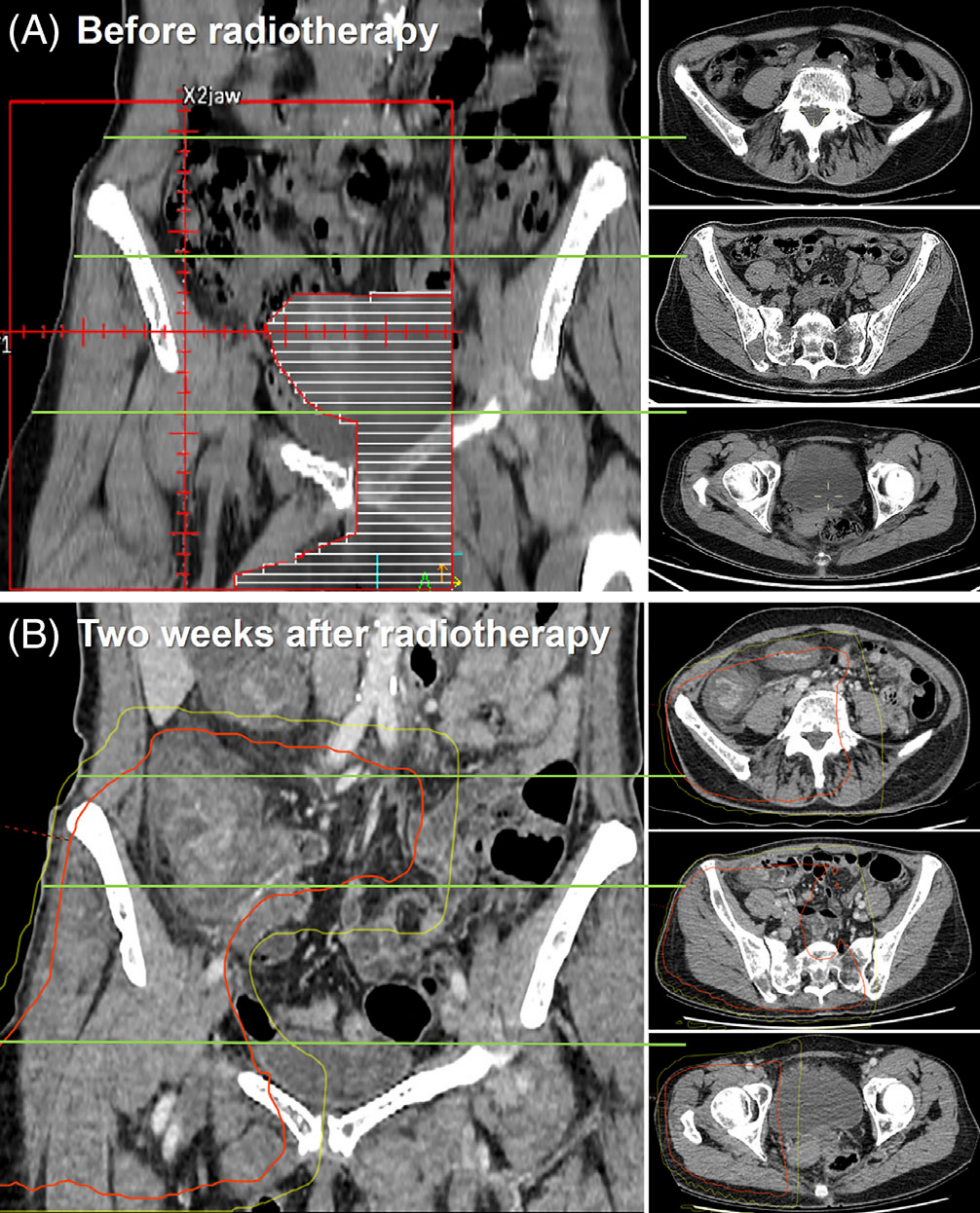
Radiotherapy field set‐up (A) and the coronal and corresponding axial diagnostic contrast‐enhanced abdominal CT 2 weeks after radiotherapy with an overlay of the 10 Gy (yellow) and 20 Gy (red) isodose lines (B). CT, computed tomography
